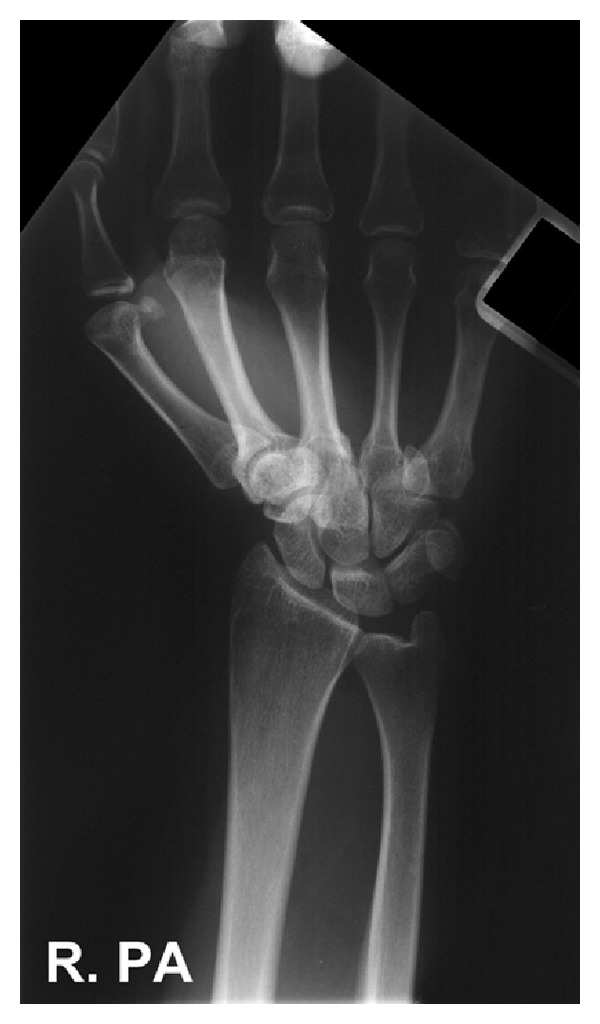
PA radiograph of the injured right wrist demonstrating normal anatomical alignment of the ulnar styloid.
